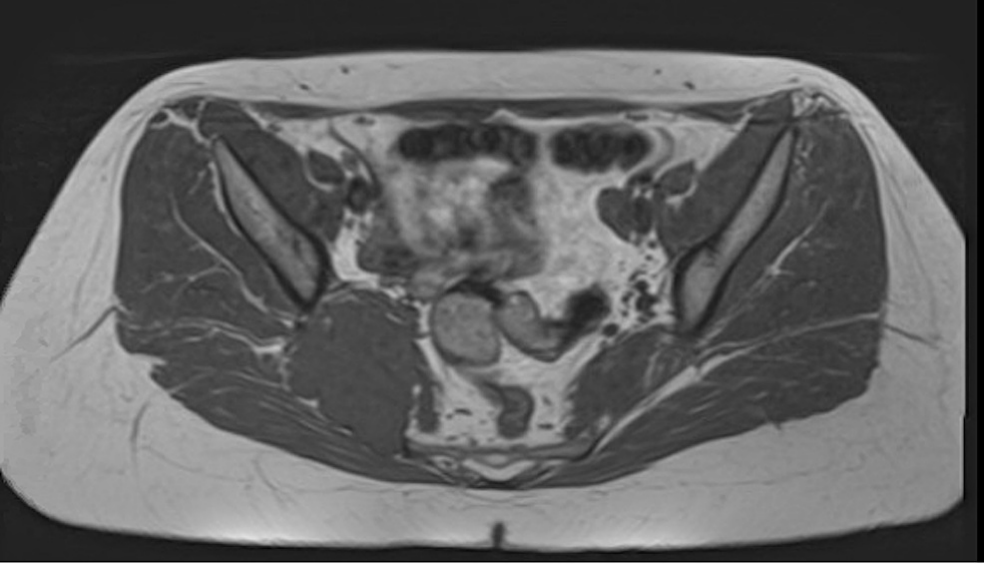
Axial view of T1. MRI showing 6 cm x 5.6 cm x 4.5 cm mass along the right ilium extending into the ipsilateral sacroiliac joint.
